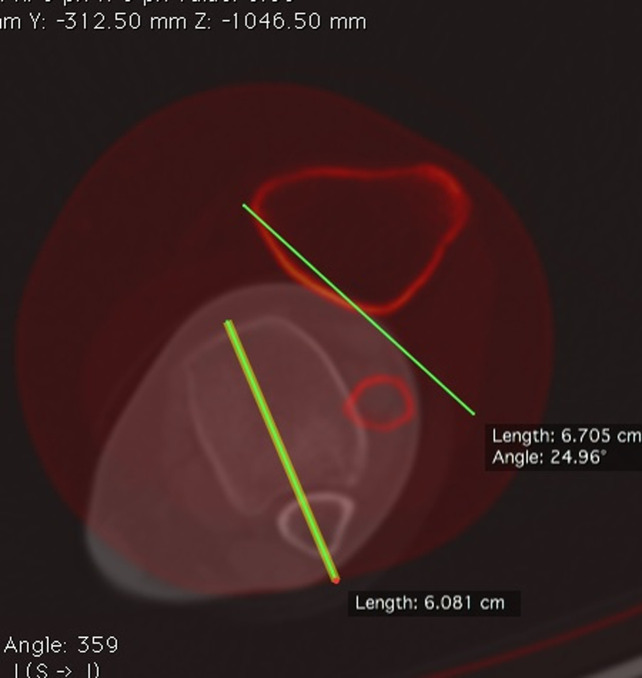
Distal tibial torsion. Superposition of two CT slices. The first slice is at the level of the tibial tubercle, and the second slice is at the malleolus. Distal tibial torsion is measured as the angle between a line joining the posterior border of the tibia at the level of the tibial tubercle and a line joining the center of the medial and the lateral malleolus.
